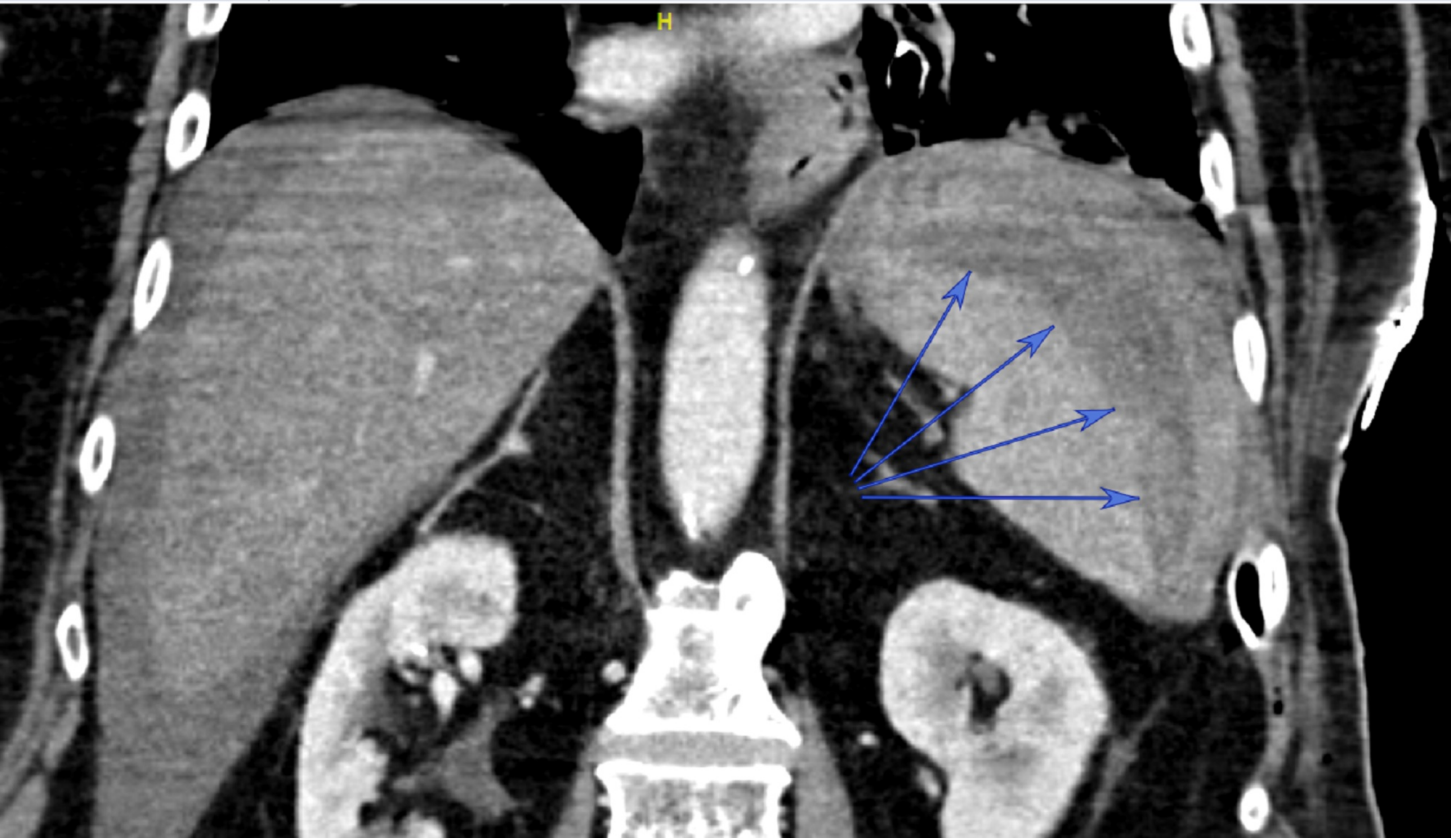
Coronal view from computed tomography scan Blue arrows identify splenic hematoma
